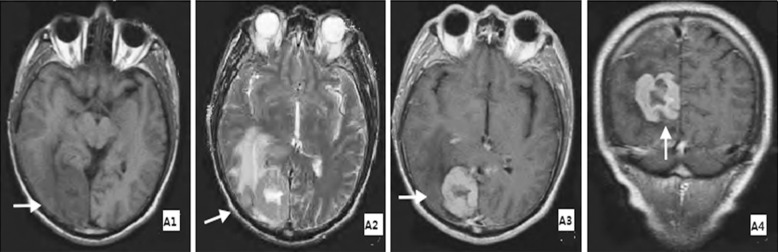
MRI performance for head of typical PCNSL patients. *Note:* A1: transverse view-T1WI, tumor with relatively low signal on right occipital lobe; A2: transverse view-T2WI, mass tumor with relatively high signal on right occipital lobe, medium edema around tumor; A3: transverse view-enhanced T1WI, significantly enhanced tumor with featured notch sign; A4: coronal view-enhanced T1WI, significantly enhanced tumor with featured notch sign. The place where the white arrow points is the location of tumor.
